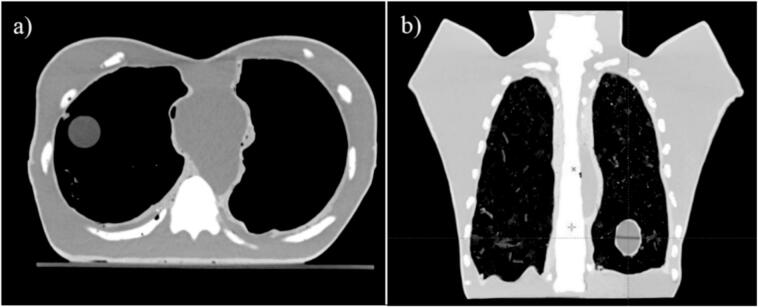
An axial CT slice with a water-filled sphere in the right lung (a) and a coronal CT slice with a movable tumor in the left lung (b).
